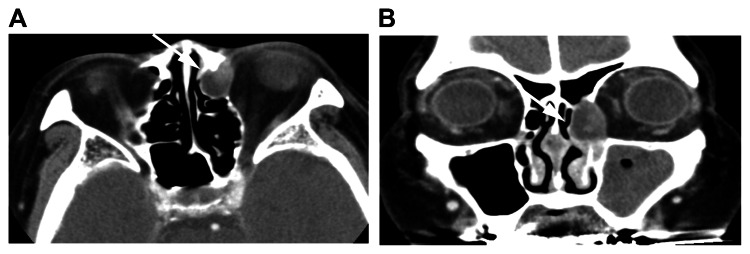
Paranasal sinus CT scan: (A) axial image and (B) coronal image A neoplastic lesion of 12×18×20 mm in size with clear margins and partial contrast enhancement is seen protruding from the lacrimal sac into the anterior ethmoid sinus. CT: computed tomography Source: Image of the patient showing the condition/feature. Informed consent for the use of this image was obtained from the patient.
